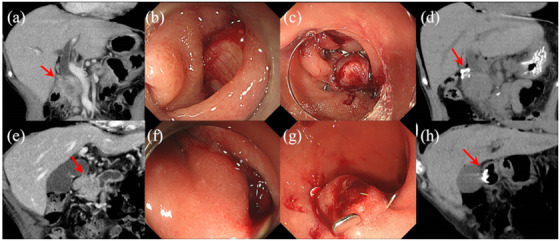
Case 1 (upper figure) and case 2 (lower figure). (a) Pancreatic head tumor on computed tomography (CT) (red arrow). (b) A large perforation, measuring approximately 13 mm in diameter, occurred in the posterior wall of the superior duodenal angle (SDA). (c) The wound filled with the omentum after placement of an over‐the‐scope clip (OTSC). (d) The OTSC detected on CT (red arrow) after the endoscopic repair. (e) Pancreatic head tumor on CT (red arrow). (f) A large perforation, measuring approximately 12 mm in diameter, occurred in the posterior wall of the SDA. (g) The wound filled with the surrounding tissue after placement of an OTSC. (h) The OTSC detected on CT (red arrow) after the endoscopic repair
